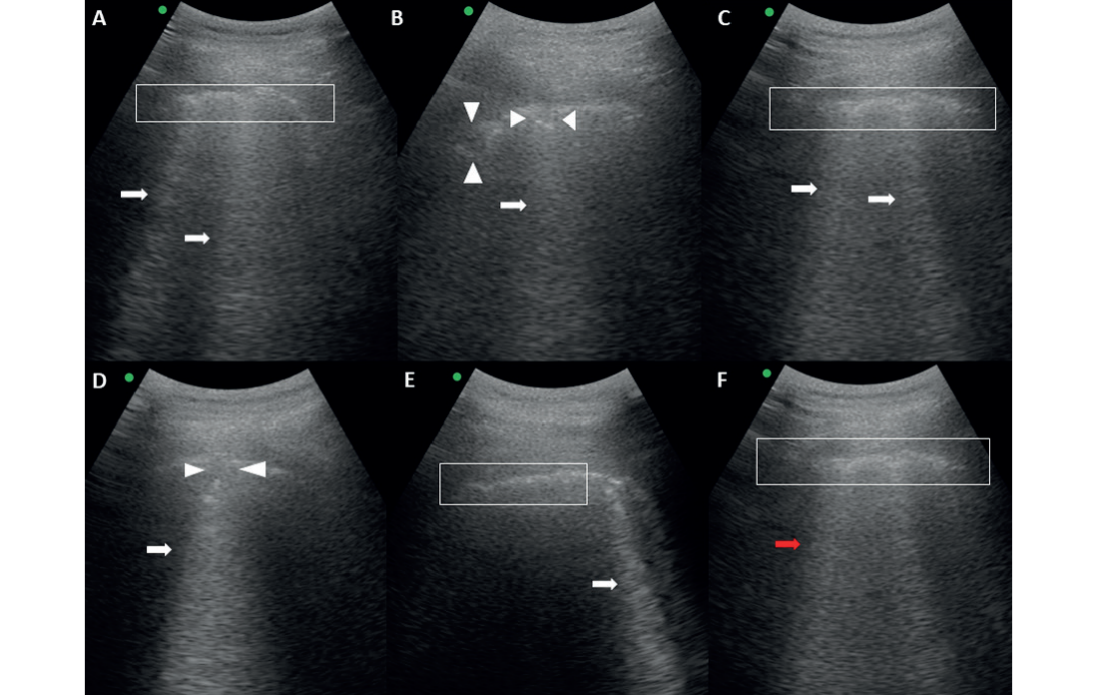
Lung ultrasound findings in a patient with coronavirus disease. Irregular plural lines (A, C, E, and F, within the white boxes); thick irregular vertical artifacts (A, B, C, D, and E, white arrows); subpleural consolidations (B and D, white arrowheads); and areas of white lung (F, red arrow) [59].
